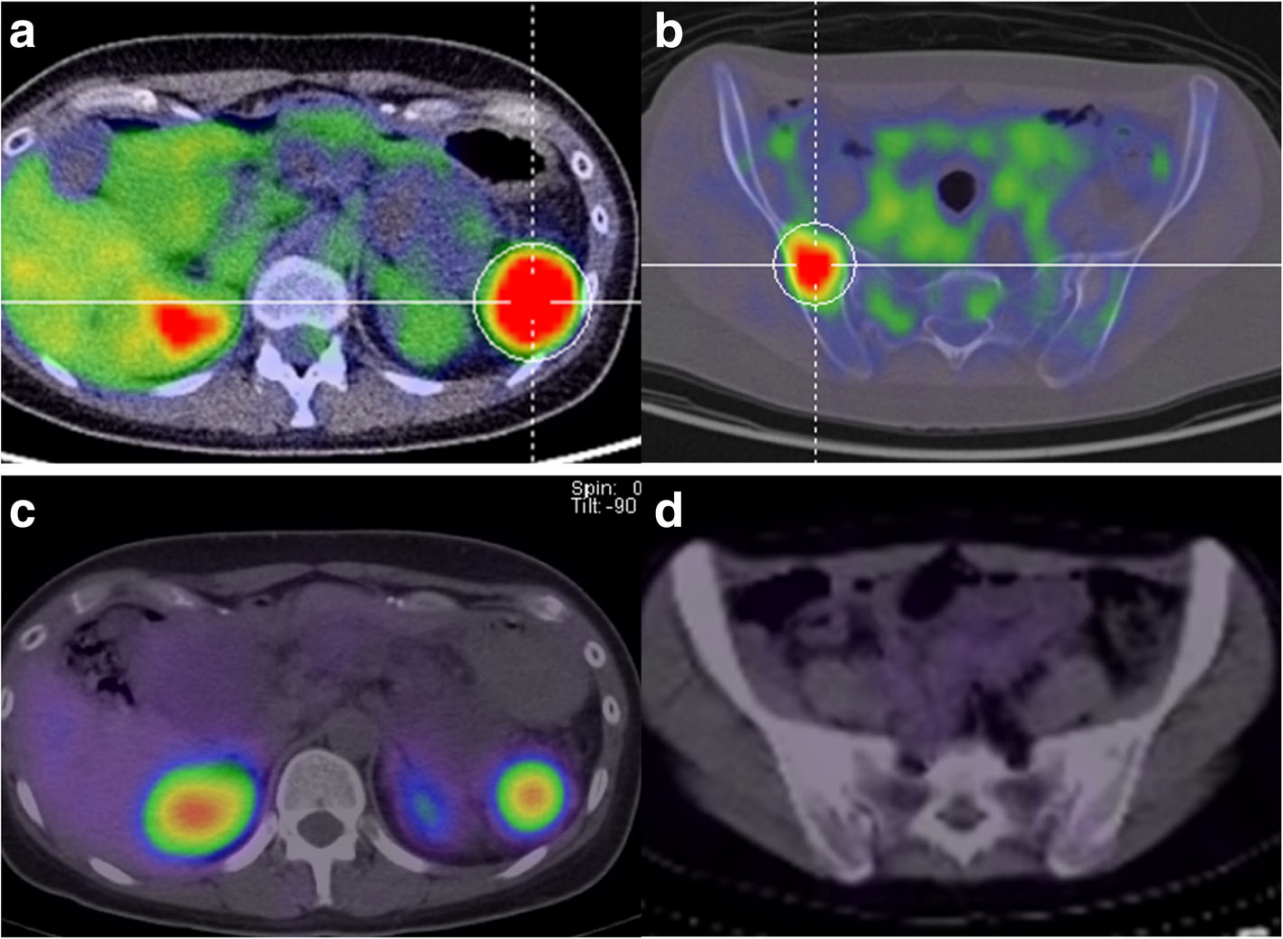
Fluorodeoxyglucose-positron emission tomography (FDG-PET) demonstrated the accumulation of FDG in a solid tumor in the tail of the pancreas (a) and the right ilium (b) with a maximum standardized uptake value of 9.5 and 9.4, respectively. Somatostatin receptor scintigraphy showed accumulation of radioactivity in the tumor in the pancreatic tail (c) but not in the right ilium (d)
